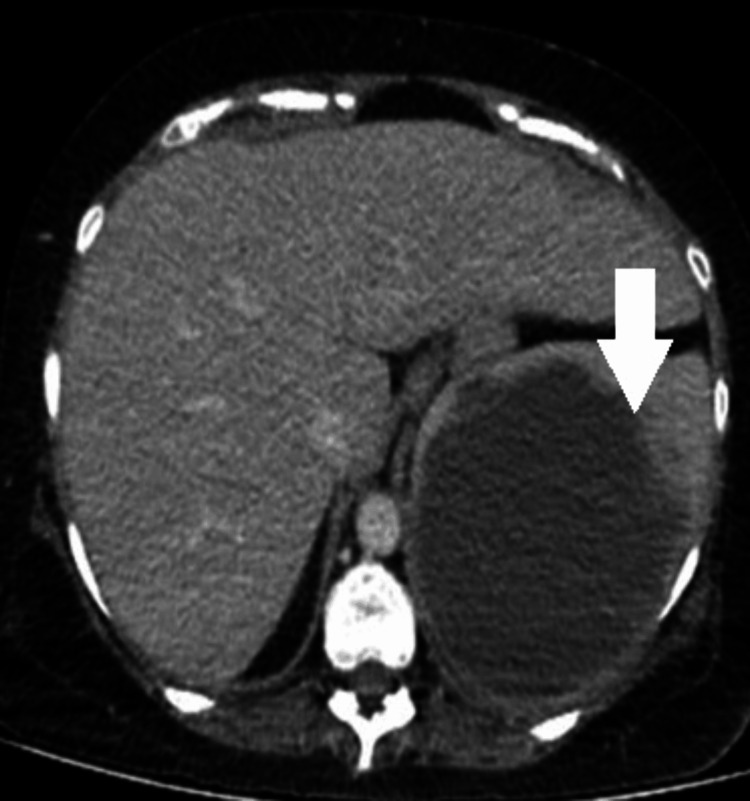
Primary splenic cyst with infection Contrast-enhanced CT abdomen showing a cystic lesion in the spleen (white arrow).
